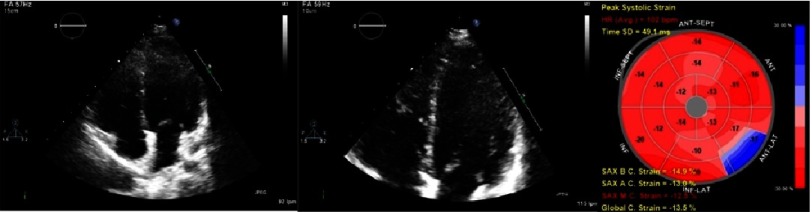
Echocardiography from a pediatric Duchenne muscular dystrophy patient. Note the left ventricle image with slight thickened interventricular septum and heterogeneous echogenicity. The last image shows regional dysfunction and decreased global circumferential strain based on speckle-tracking.
